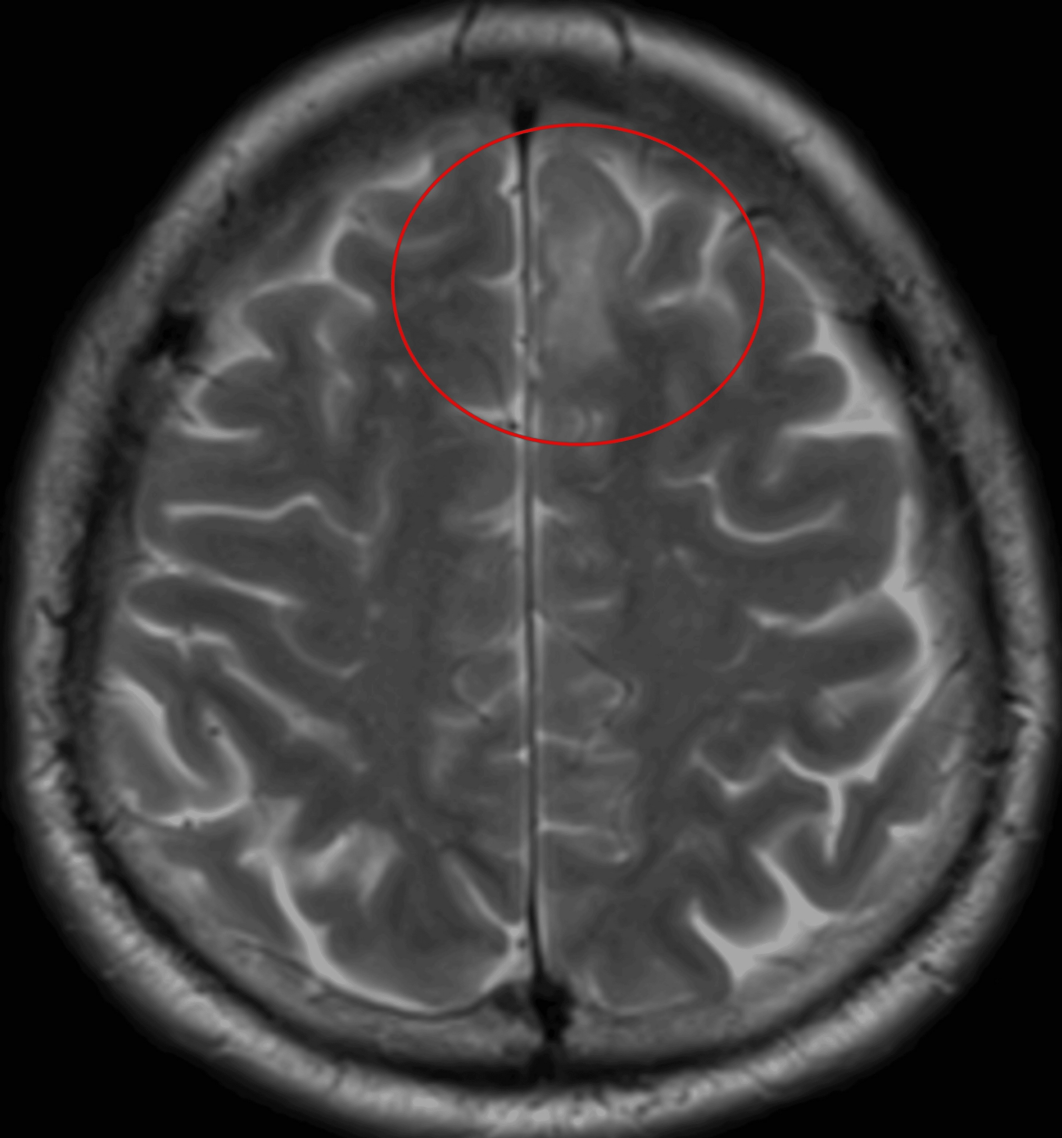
MRI revealed a tumorous lesion in the right frontal lobe.
